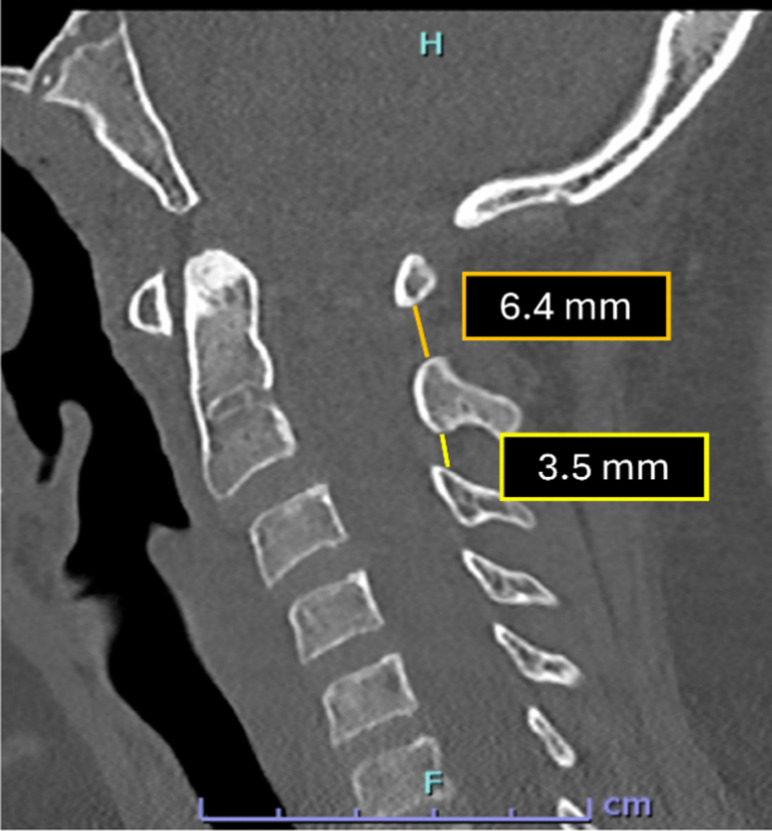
Sagittal CT of the cervical spine in a 7-year-old child demonstrating interspinous distance measurements at the C1–2 and C2–3 levels. Here, the measurement is obtained by dividing 6.4 mm (C1-C2 distance) by 3.5 mm (C2-C3) measuring 1.8 (normal ratio <2.5). Measurements are obtained in the midline, extending from the posterior cortex of the inferior spinous process to the superior tip of the adjacent superior spinous process, following reference thresholds originally established on lateral radiographs
